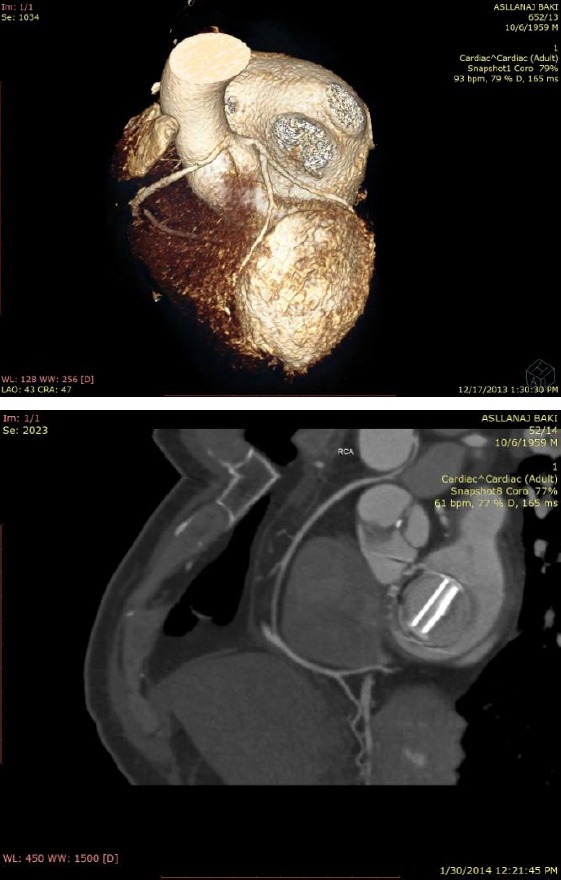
CT scan and 3D reconstruction of the patient before (up) and after surgery (down)*.
